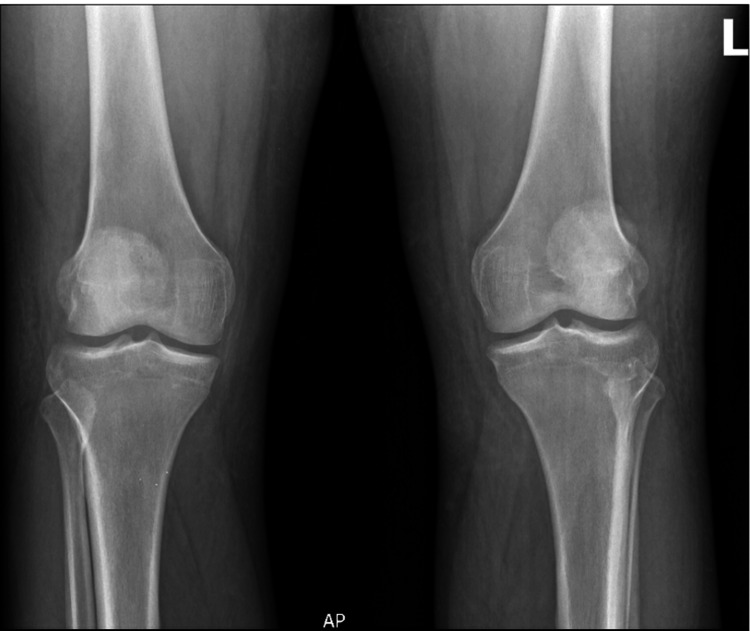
Postoperative anteroposterior (AP) radiograph of bilateral knees AP radiograph demonstrates preserved joint spaces bilaterally with no evidence of fracture or degenerative changes.
